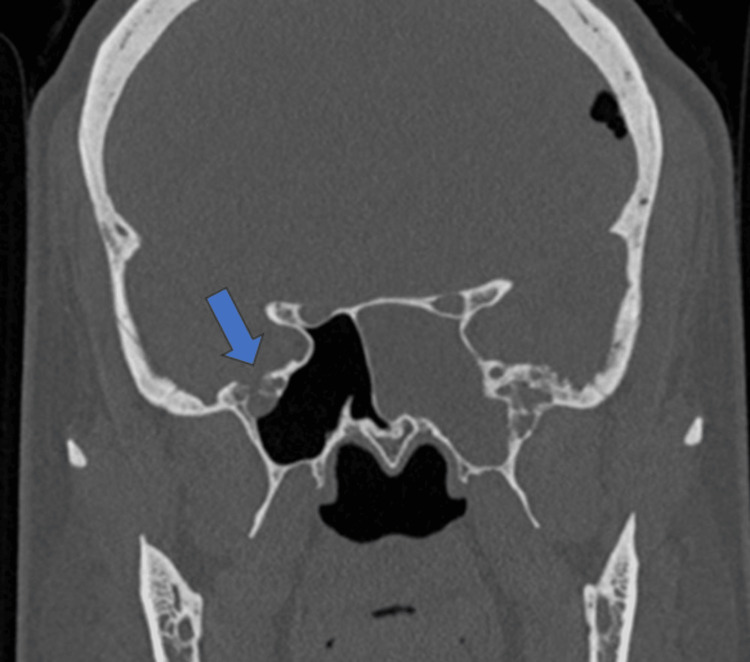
Coronal CT section at the level of sphenoid sinuses The image shows a small focal defect (blue arrow) in the right lateral recess of the sphenoid sinus with mild adjacent fluid opacification.
